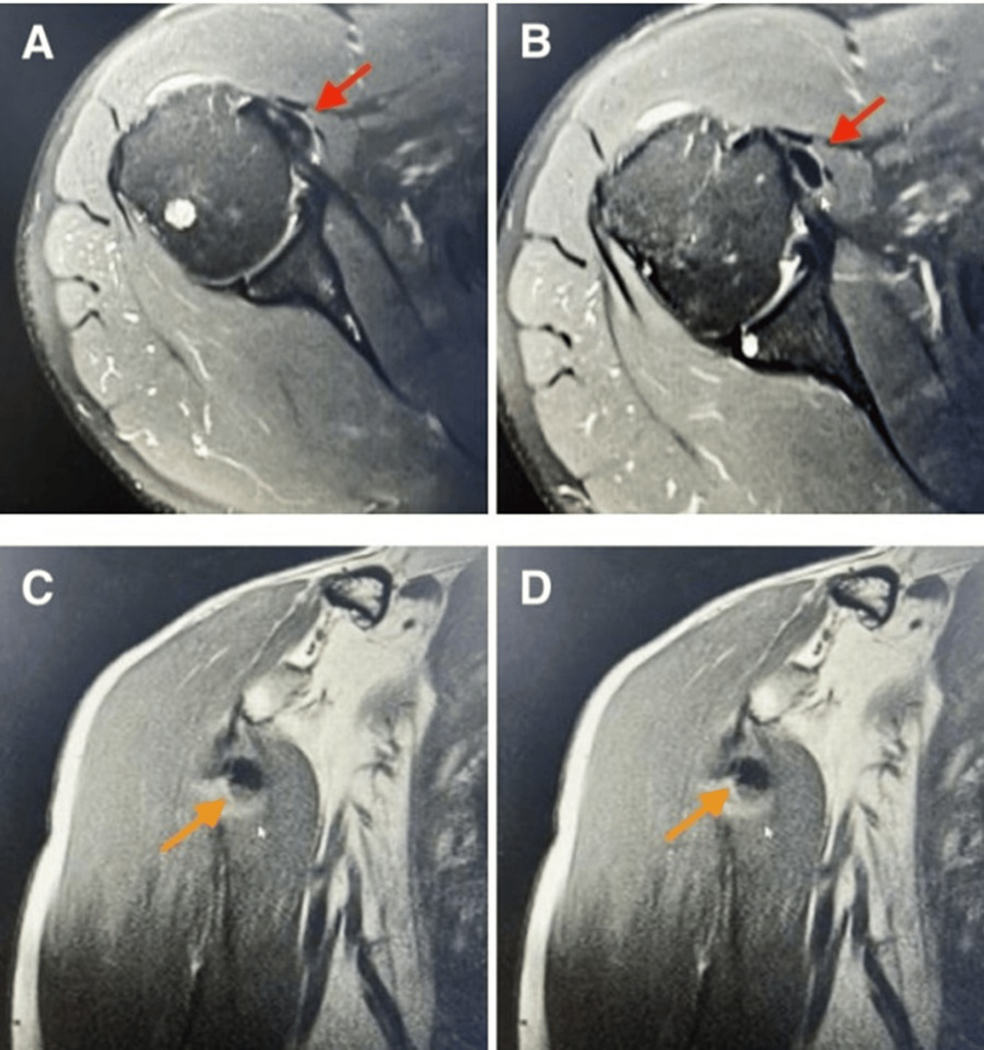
Right shoulder MRI (A and B) Axial cuts showing a large calcium deposit found within the substance of the subscapularis tendon measuring roughly 9 × 5 mm (red arrow). (C and D) Coronal cuts showing that the calcific deposit within subscapularis tends to be larger (orange arrow).
